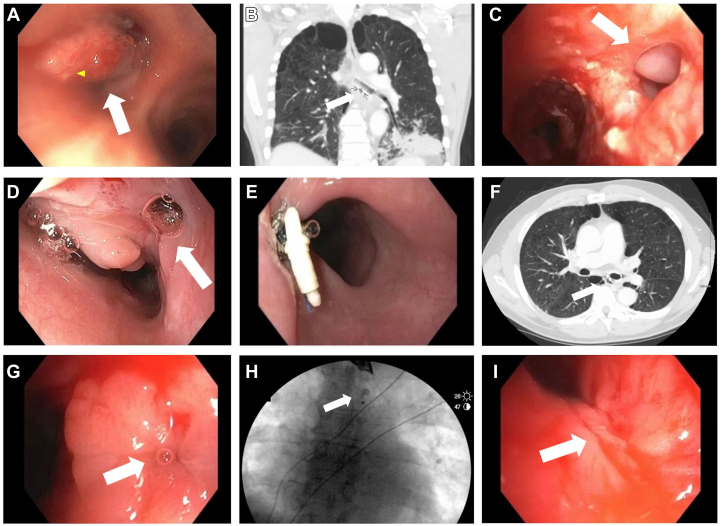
(A) Pretreatment rigid bronchoscopy was used to diagnose the original left mainstem bronchus tumor indicated by both yellow and white arrows. (B) A screenshot of a coronal section of the chest computed tomography scan showed extraluminal air (arrow) adjacent to left mainstem bronchial stent. (C) Bronchoscopy showed the 10-mm bronchoesophageal fistula (BEF; arrow) in the medial wall of the left mainstem bronchus just distal to the carinal bifurcation. (D) Esophagogastroduodenoscopy also showed the 10-mm BEF (arrow) 30 cm from the incisors. (E) X-Tack suturing was used for the initial closure of the fistula. (F) A screenshot of an axial section of the 5-month surveillance chest computed tomography scan showed the persistent fistulous tract (arrow). (G) Repeated bronchoscopy showed the persistent 4-mm fistula opening (arrow). (H) Prolaryn gel was injected into the fistula and confirmed on fluoroscopy (arrow). (I) Repeated bronchoscopy 2 months after showed completely healed BEF (arrow).
